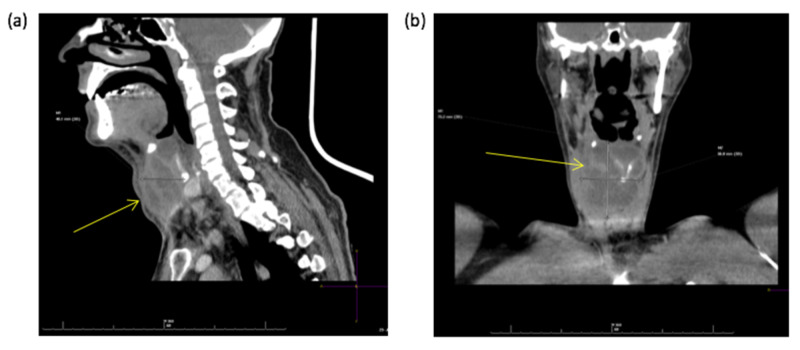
Sagittal (a) and coronal (b) sections of contrast-enhanced CT scan demonstrating a multiloculated collection (red arrows) in the midline of the anterior neck, measuring 7.5 cm × 5.6 cm × 4 cm.
